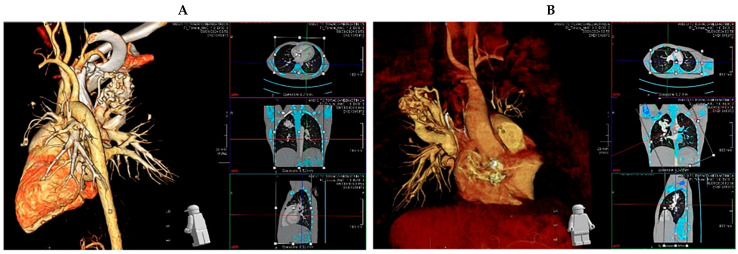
Frontal (A) and lateral (B) views from chest angio CT showing the largest AVM in the upper lobe of the right lung.
